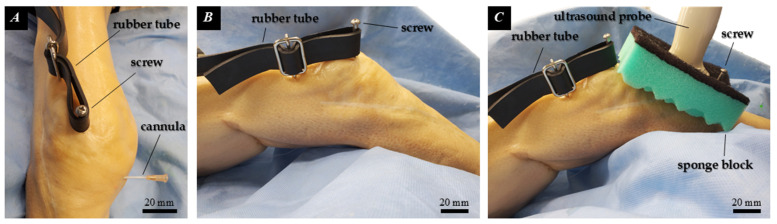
A Thiel-embalmed right knee at 45 knee flexion. (A) Anterior view; (B) Lateral view; (C) Lateral view demonstrating ultrasound probe application.
